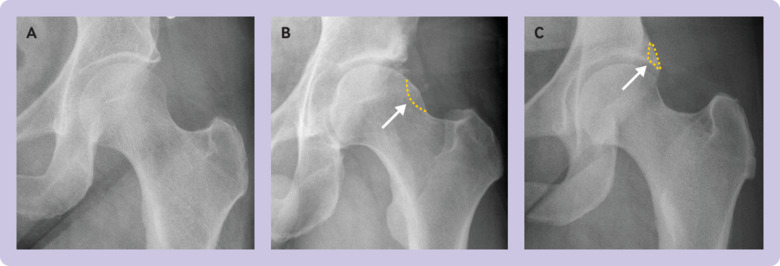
Anteroposterior radiographs showing (A) normal morphology, (B) cam morphology, and (C) pincer morphology. Dashes represent abnormal morphology that predisposes to femoroacetabular impingement, and which is excised with a burr during arthroscopic surgery to prevent impingement
